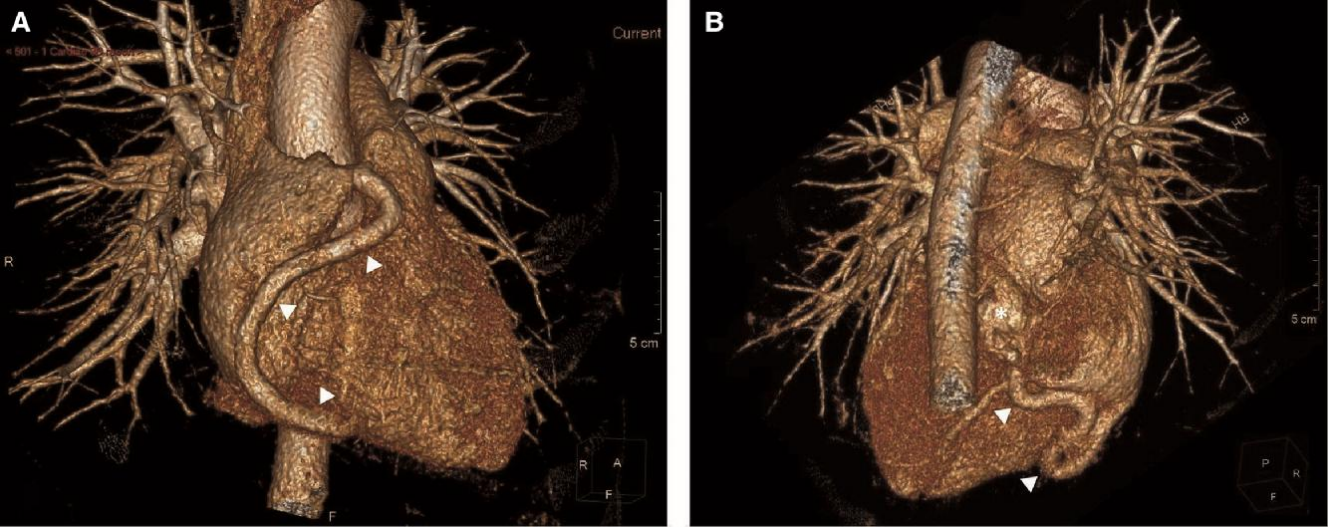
Three-dimensional image reconstruction of the computed tomography coronary angiogram. (*A*) Right anterolateral view showing the course of the right coronary artery, which was dilated (arrowheads). (*B*) The dominant right coronary artery (arrowheads) was tortuous and followed a posterior–inferior course, where it finally abutted the coronary sinus (asterisk).
